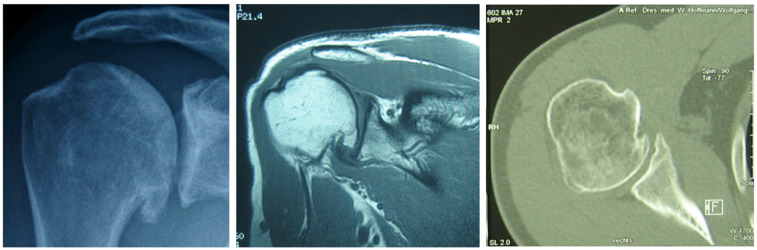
Imaging methods for evaluating dislocation arthropathy. Examples of conventional radiographs, MRI, and CT scan showing OA.
